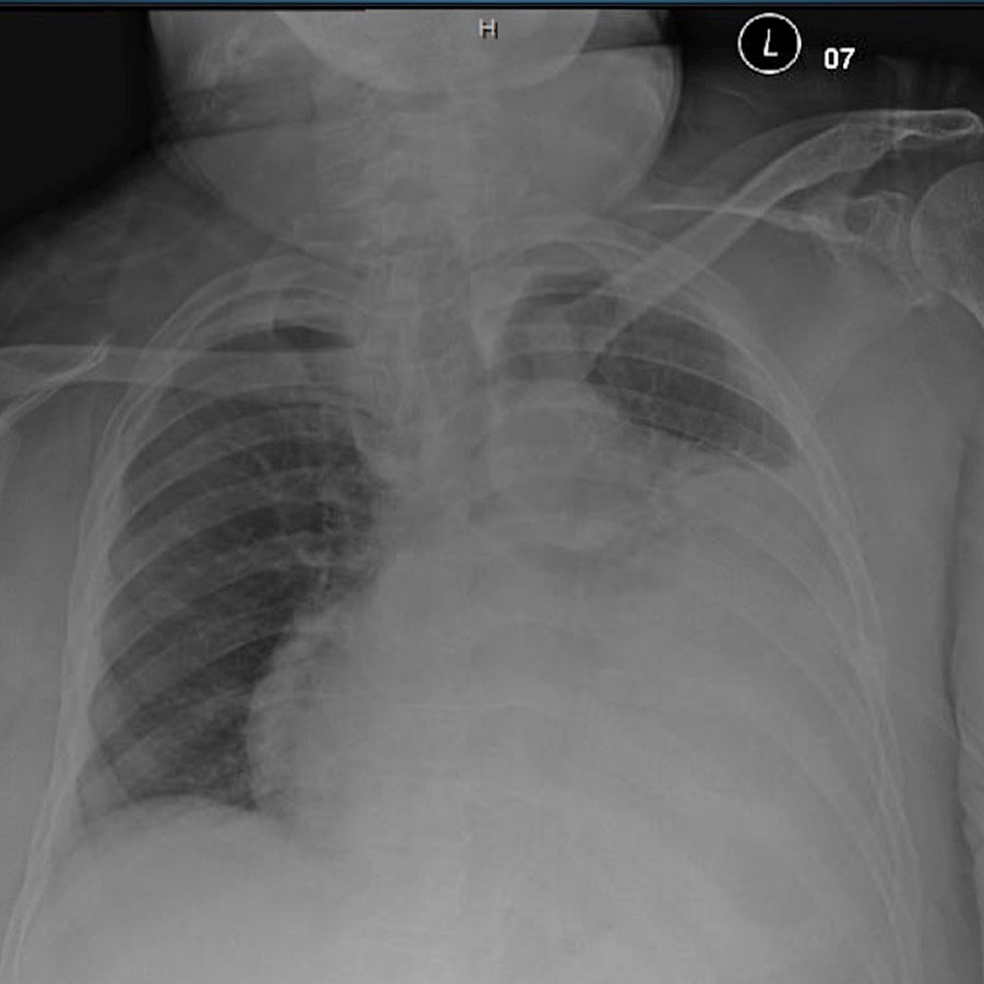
Admission chest radiography showing enlarged cardiac silhouette and congested lung fields
